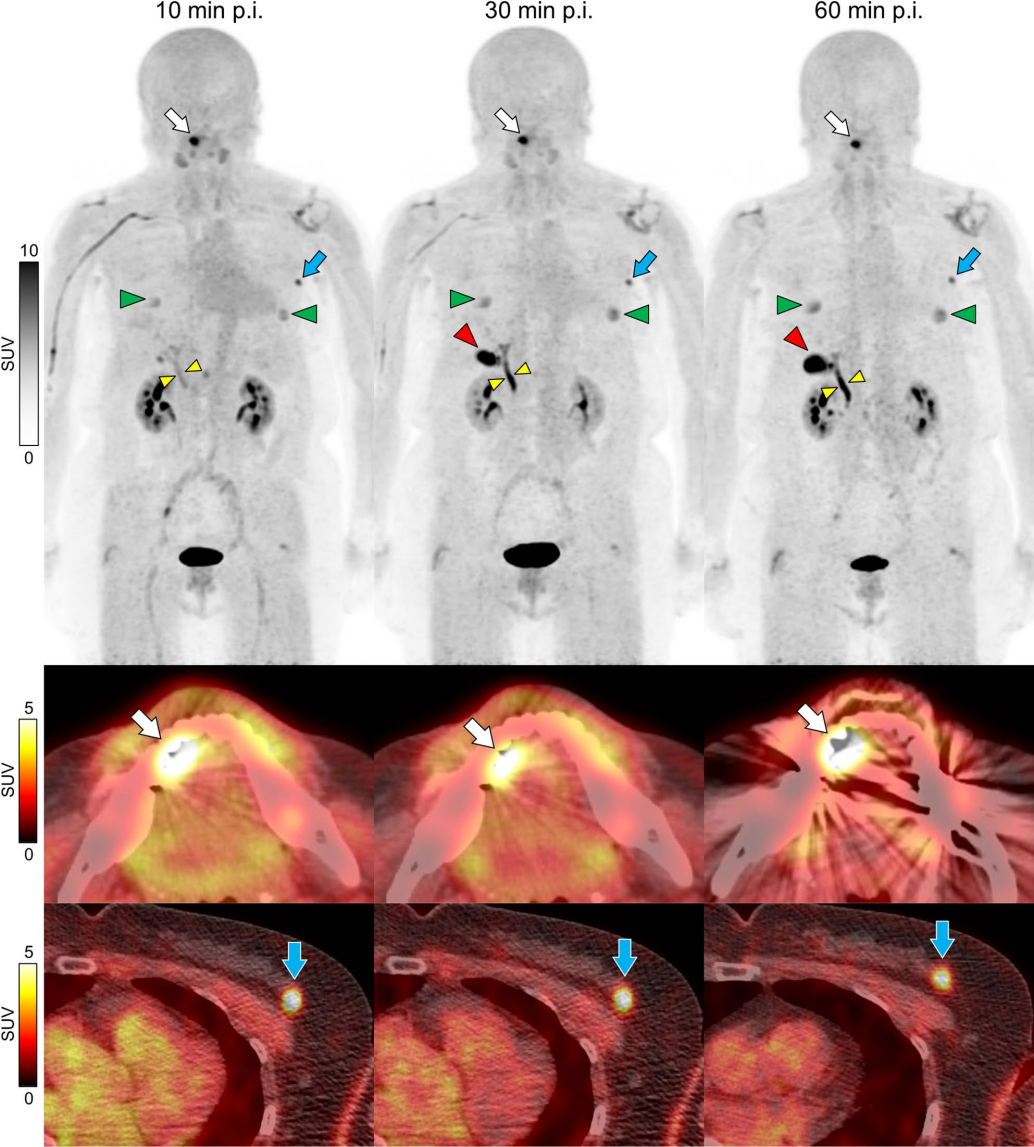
Representative case of three time point [^18^F]FAPI-74 PET/CT imaging Maximum intensity projection (MIP) images and axial [^18^F]FAPI-74 PET/CT fused images of 70-year-old female with tongue and breast cancers. Uptake in most normal organs such as the liver, spleen, and blood pool decreased over time. Uptake in the gallbladder (red arrowheads), bile duct (yellow arrowheads), and nipple (green arrowheads) gradually increased. There was an uptake of tongue cancer on the right side of the oral cavity (white arrows). Uptake in the left breast was incidentally detected in breast cancer (blue arrows). Grayscale indicates standardized uptake values (SUV) of the images. p.i.: post injection
